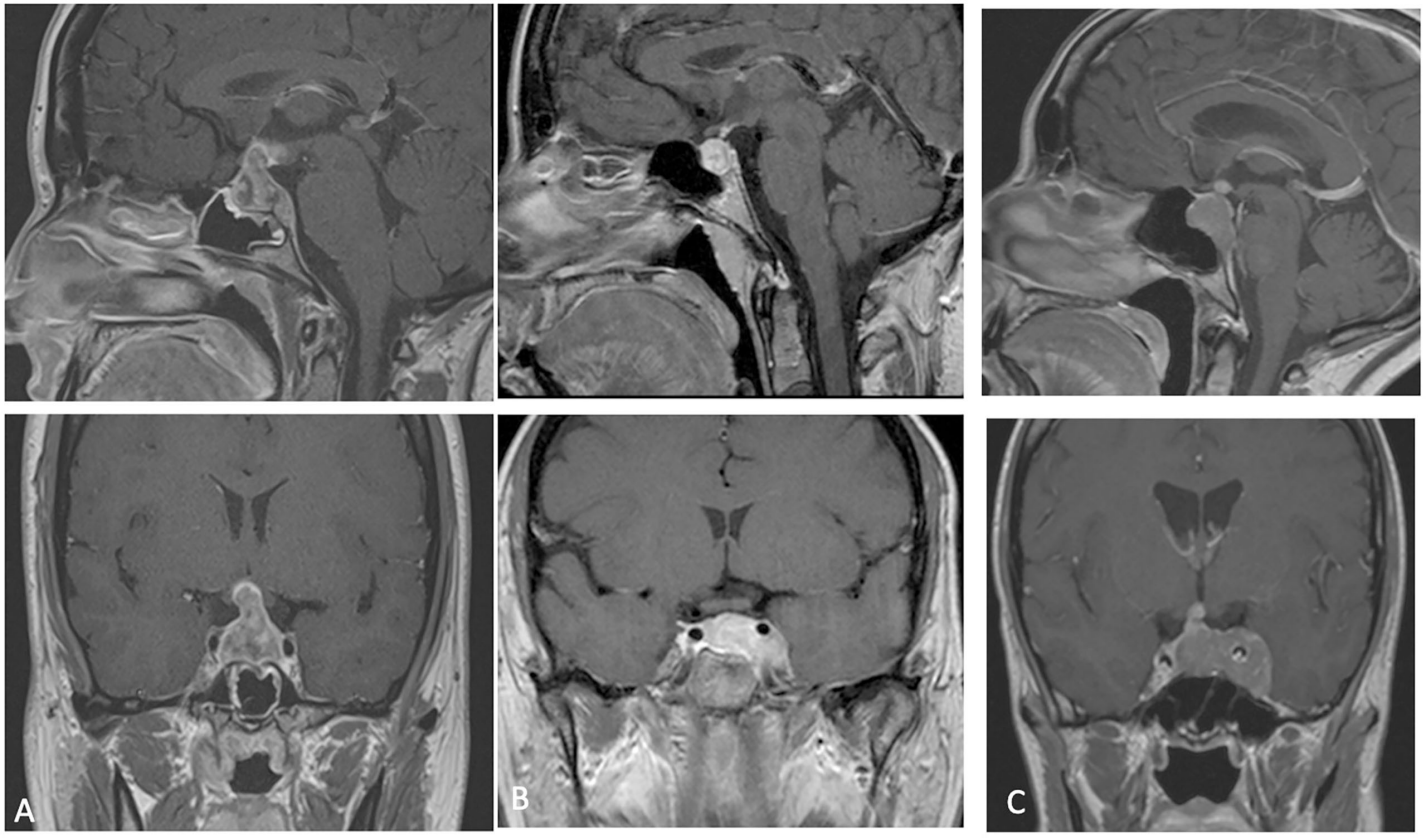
(A–C) preoperative MRI (cases 1-2-3) showing a lesion with heterogeneous post-contrast enhancement and intra supra and para-sellar extension.
